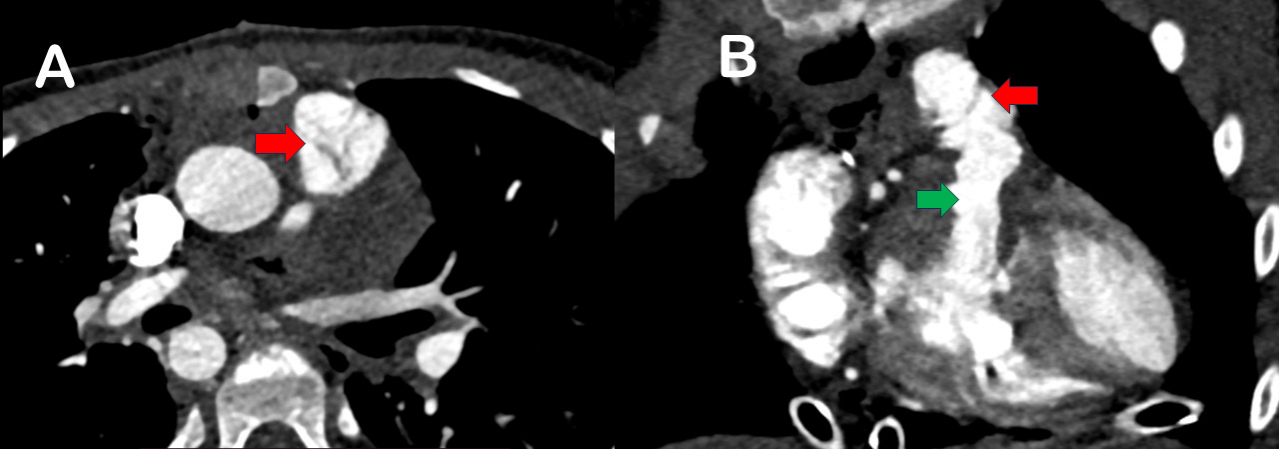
Computed tomography images (A and B) depicting pericardial valve
